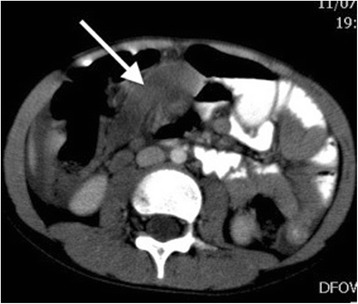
Computed tomographic scan shows a fusiform, nonenhancing solid mass situated between the right hemiabdomen and the midline (*arrow*)
